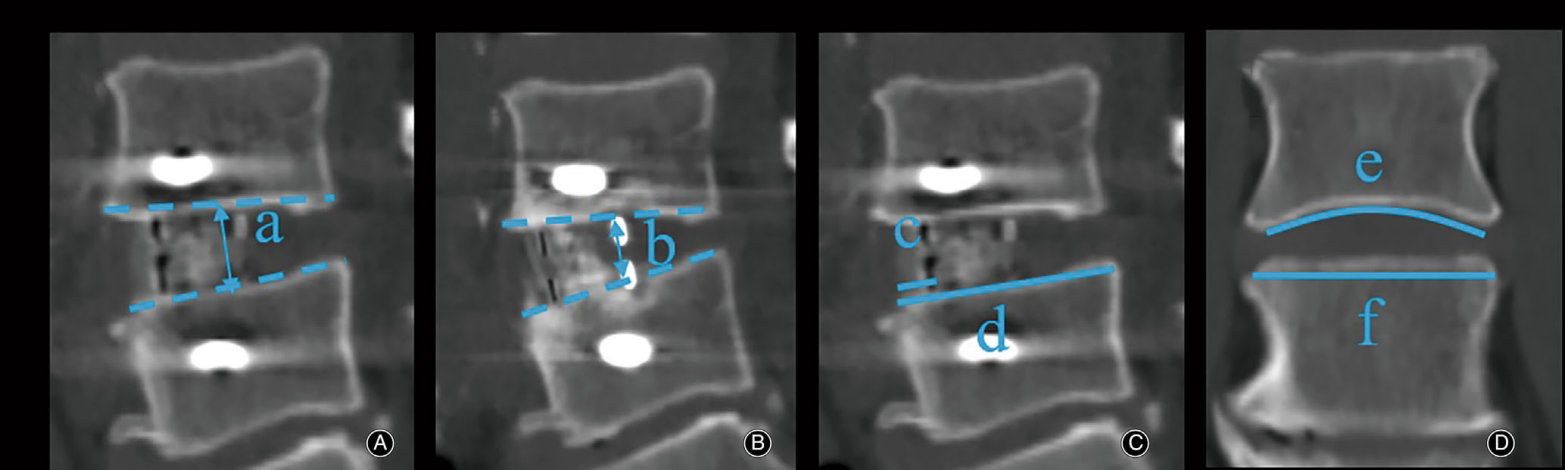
Measurement of disc height on 2D CT midsagittal plain reconstruction. (a, b) The vertical distance between the midpoint of the superior and inferior endplates; (c) the distance between the anterior marker of the cage and the anterior edge of the vertebral body; (d) the distance between the anterior edge of the vertebral body and the posterior edge of the vertebral body; (e) concave endplate morphology; (f) flatendplate morphology; (A) disk height measured 1 day postoperatively; (B) disk height measured at the last follow‐up; (C) cage position; (D) endplate morphology.
